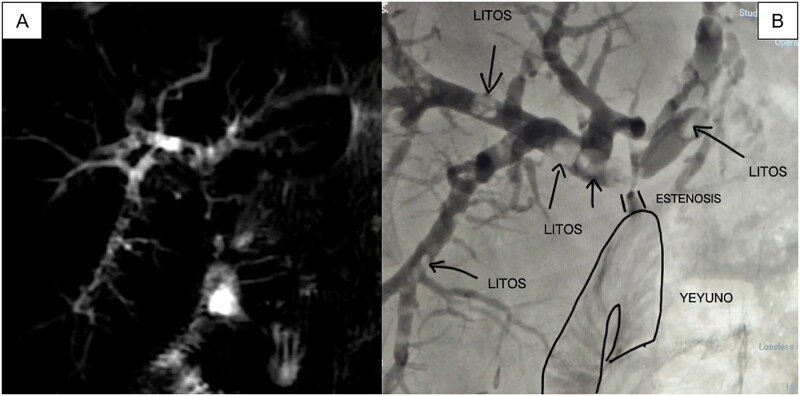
(A) MRI showing multiple filling defects in the right and left main branches, as well as stenosis at the level of the HJA; (B) Initial cholangiography obtained by puncturing the peripheral branch of segment VI with a 21-gauge needle confirms the same findings as the MRI.
